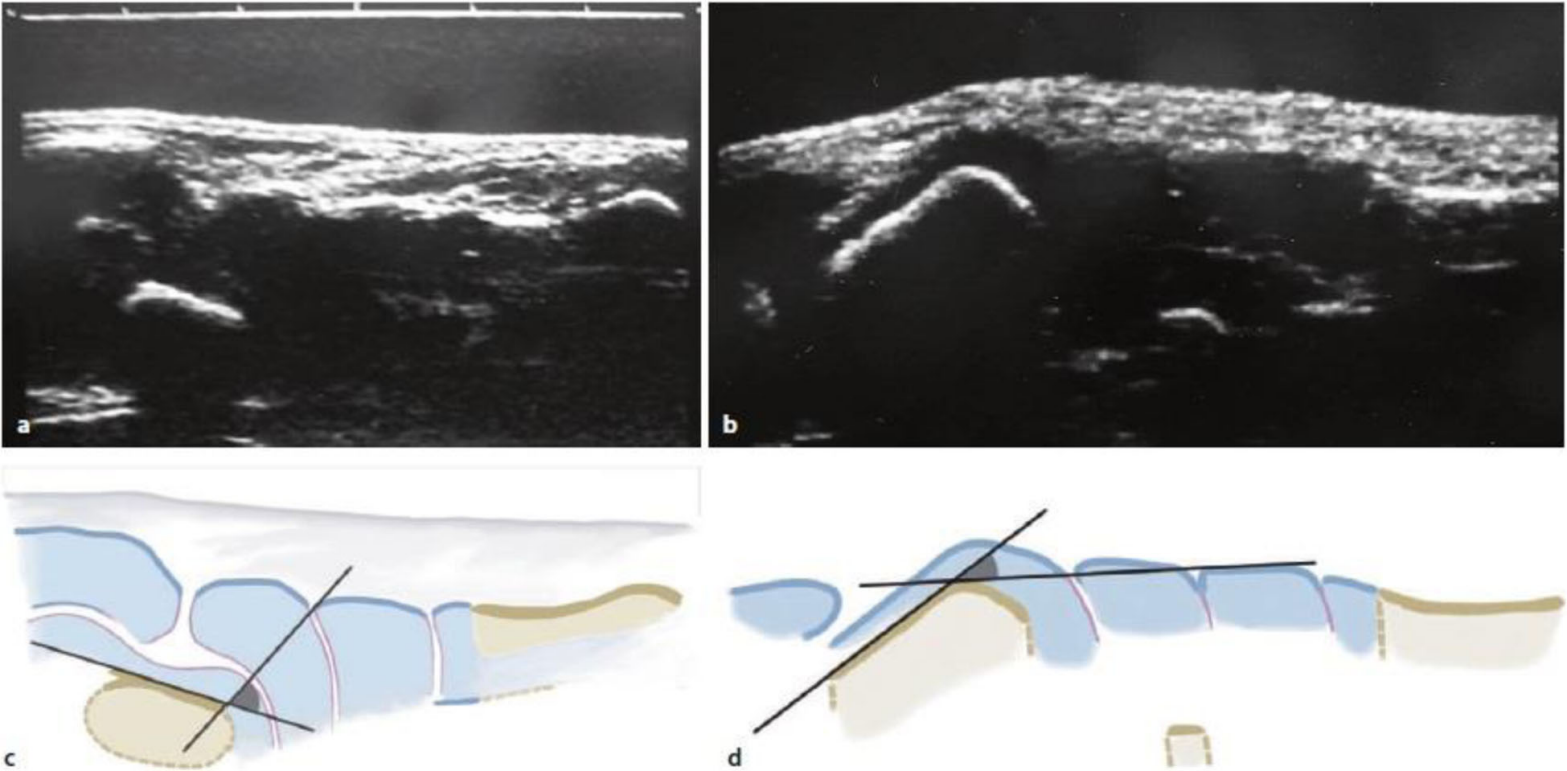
Sonographic transverse section of an uncorrected clubfoot at the age of 5 months (a, c) with a TnCE angle of approx. + 75 ° and healthy contralateral side (b, d) with TnCE angle of approx. -30 °
